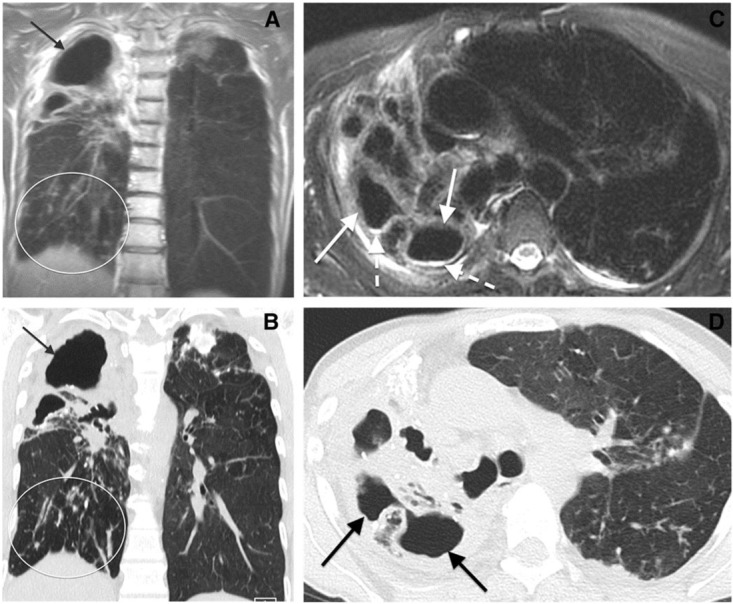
Comparison of chest MR to CT imaging for assessment of *Mycobacterium avium* complex lung infection. Cavitary Mycobacterium avium complex (MAC) pneumonia in a 64-year-old woman. (A) Coronal MR and (B) coronal computed tomographic (CT) images show cavitary disease (arrows) and traction bronchiectasis in the right upper lobe. Subcentimeter nodules (oval) are present in the right lower lobe. (C) Axial MR and (D) CT images of the upper lungs show cavitary disease (solid arrows) and traction bronchiectasis in the right upper lobe. Reproduced with permission from Chung et al. (2016).
